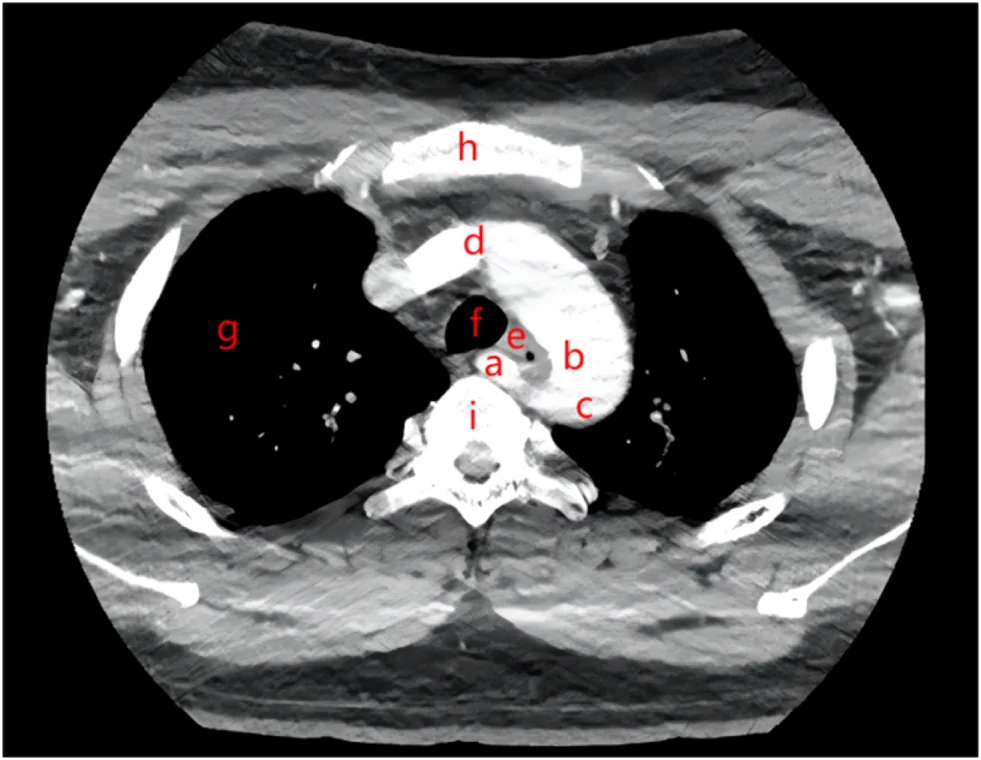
Preoperative contrast-enhanced CT of the neck demonstrates that the right subclavian artery courses behind the esophagus and is directly connected to the aortic arch. (A) Aberrant right subclavian artery; (B) Aortic arch; (C) Descending aorta; (D) Ascending aorta; (E) Esophagus; (F) Trachea; (G) Lung; (H) Sternum; (I) Vertebrae.
